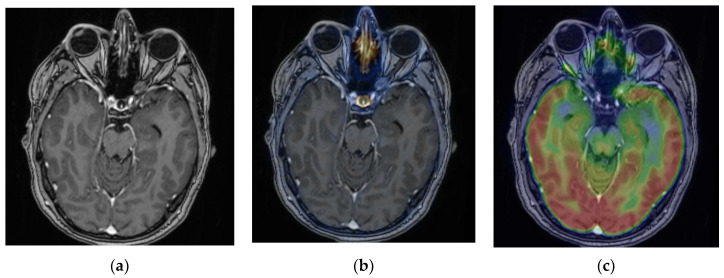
Case 7. Axial slices of T1W1 gadolinium (a), ^68^Ga-DOTATOC fused PET-MRI (b), and ^18^F-FDG fused PET-MRI (c) showing low DOTATOC (SUV_max_ = 3.6 for the lesion, and SUV_max_ = 10.2 for the pituitary) and moderate FDG uptake in the left optic nerve cisternal segment enhanced lesion.
